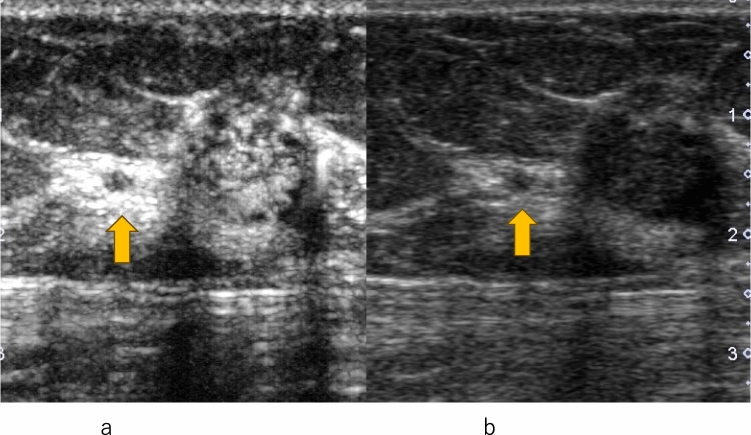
Invasive ductal carcinoma. a Contrast-enhanced ultrasound of a small hypoechoic daughter lesion (arrow) shows heterogeneous enhancement. b Conventional ultrasound shows a mass with a small hypoechoic daughter lesion (arrow)
